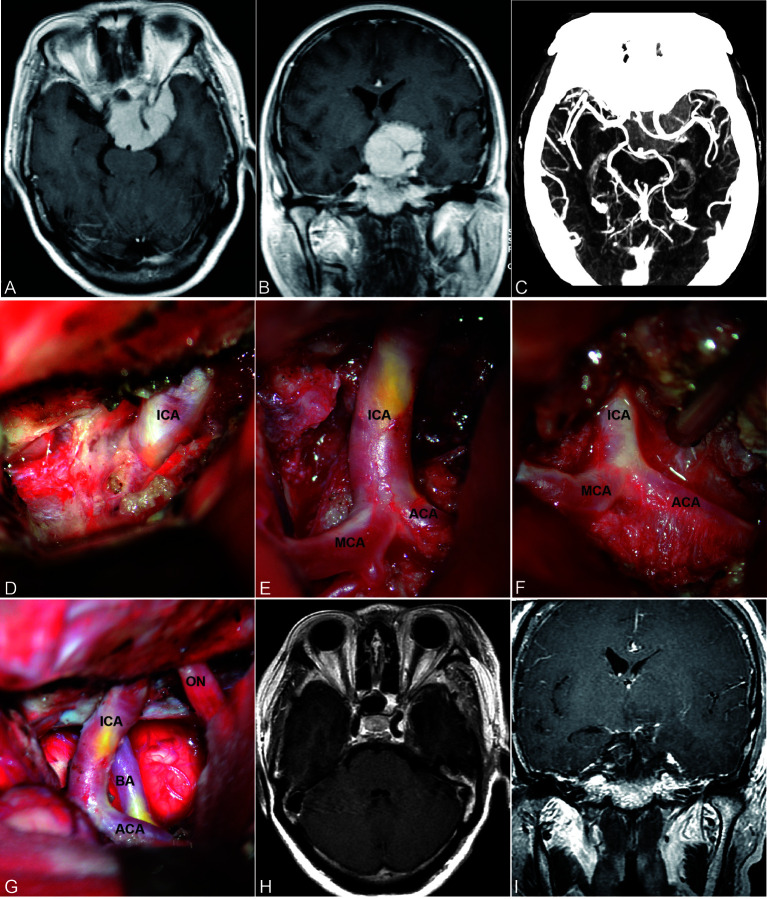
Contrast-enhanced MRI and CTA images of ACM (A–C) preoperative; H, I postoperative) and steps of bidirectional dissection technique (D–G). Intradural localization of the ICA (D) and dissection along the course of ICA and its branches (E, F) to achieve total resection while preserved the ICA and its branches (G).
